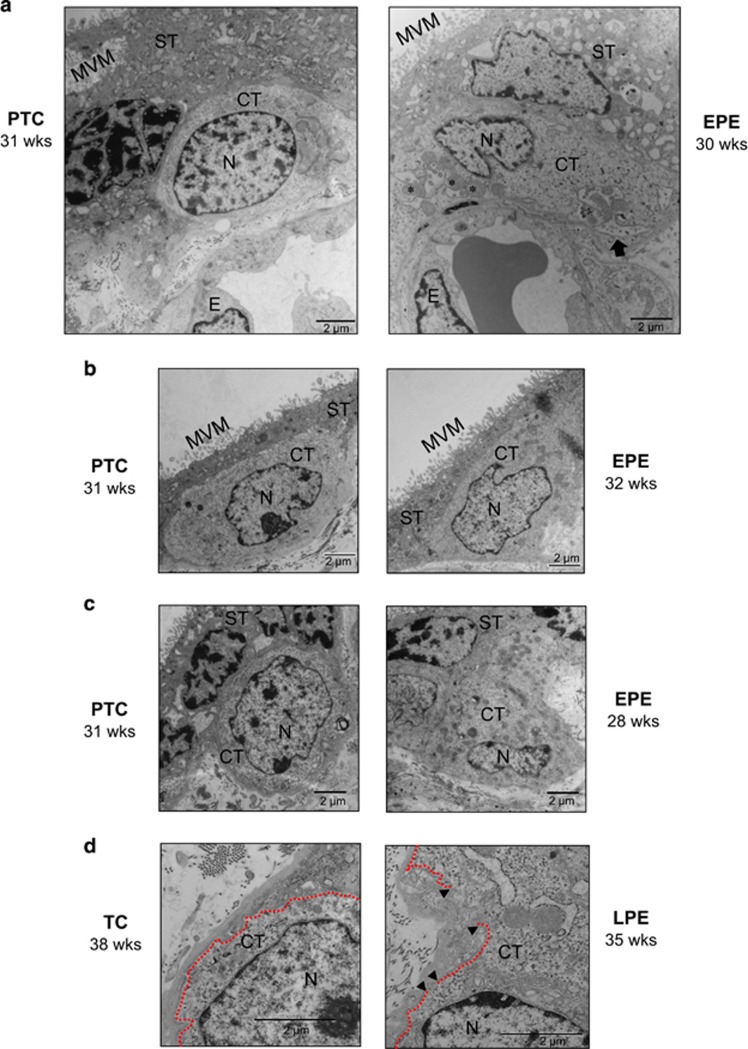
Preeclampsia is associated with necroptotic cytotrophoblast cell morphology. (a) TEM analysis of PTC and PE placental tissue sections. Necrosis morphology was identified based on swollen mitochondria (indicated by asterisks), loss of cytoplasmic electron density, and membrane disruptions. An arrow designates evidence of mitophagy in the PE sample. (b and c) Representative TEM images of cytotrophoblast cells from E-PE and PTC placentae, showing loss of chromatin organization (chromatin decondensation), aberrant nuclear morphology and loss of cytoplasmic electron density in PE samples compared with PTC. (d) Representative TEM images from L-PE and TC placentae showing discontinuous cell membrane of necrotic cytotrophoblasts from PE placentae, as occurs in the final stages of necrosis. This is depicted by gaps (indicated by black arrowheads) in the plasma membrane (depicted with dashed line). CT, cytotrophoblast; E, endothelial cell; MVM, microvillous membrane; N, nucleus; ST, syncytiotrophoblast. Scale bars=2 *μ*m
